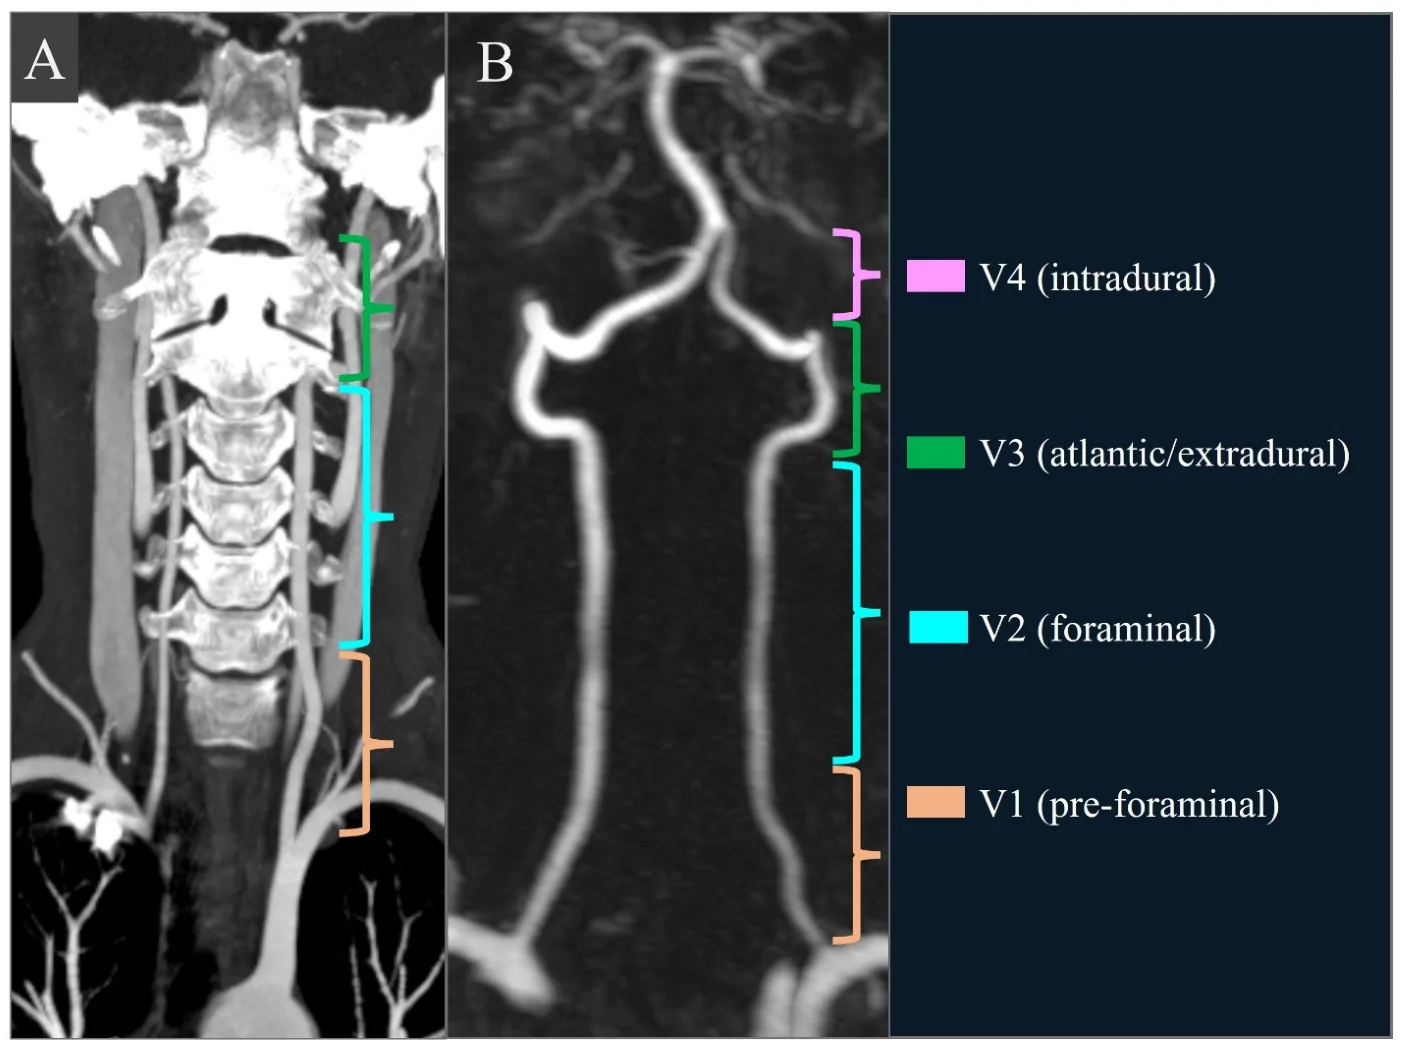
Anteroposterior projection CTA maximum intensity projection (MIP) (A) and MRA MIP (B) images showing the 4 segments of the VA.
